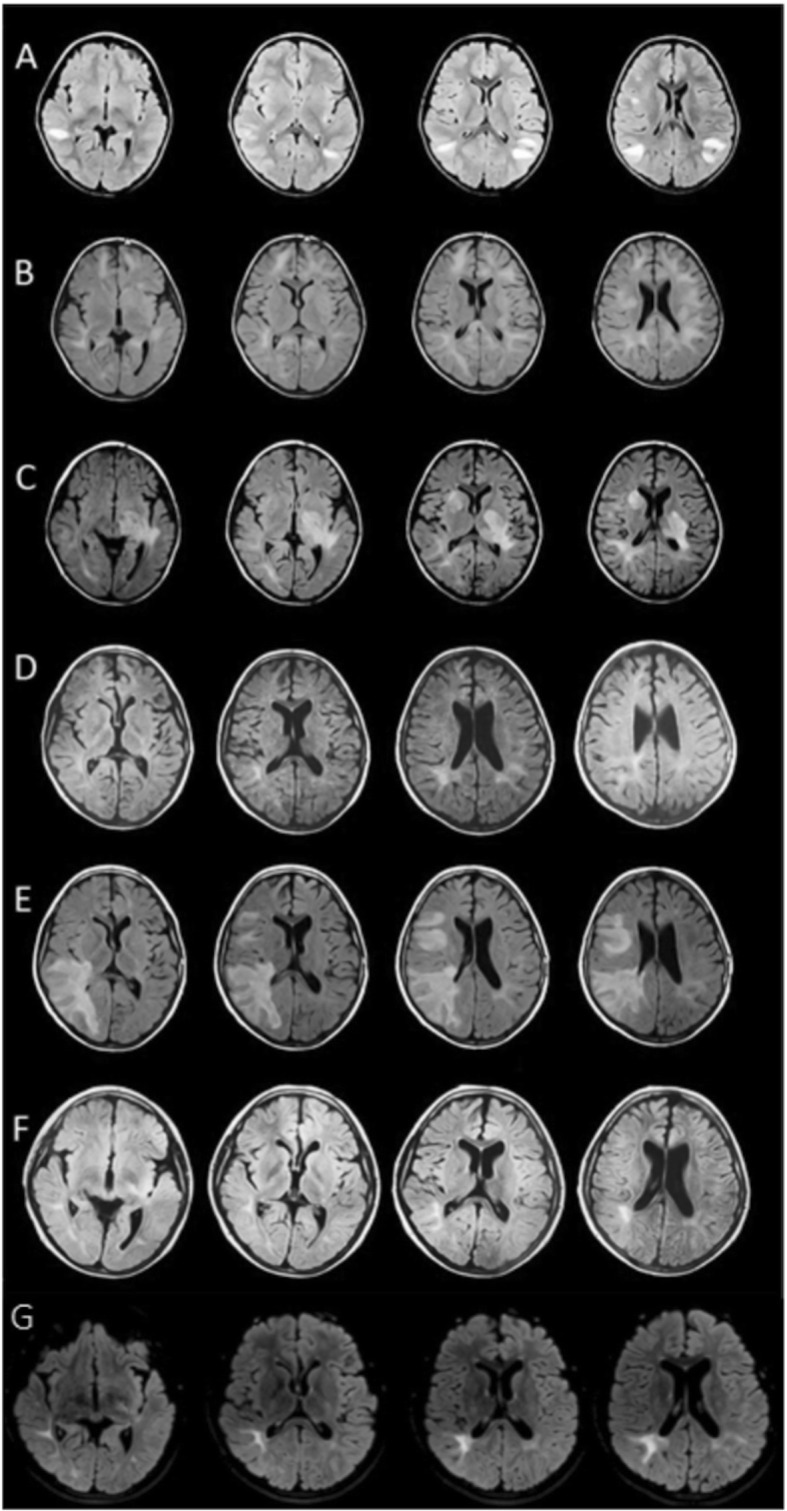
Fluid-attenuated inversion recovery T2 sequences showing multiple hyperintense lesions through the years. a 2008: Multiple injuries, mainly subcortical in temporal and occipitotemporal gyrus, and in the white matter of the corona radiata and semioval center. Lesions are enhanced after contrast. b 2010: Frontal subcortical injuries. Periatrial and parahippocampal lesions. There is a persistence of white matter lesions, and there are new infratentorial lesions. c 2011: Persistence of the periatrial lesions and left midbrain lesion. d 2013: Persistence of periatrial lesions and two small and new lesions in the basal ganglia infratentorial lesions. e 2014: New appearance of lesions in the cortex. Frontal and right superior temporal subcortical lesions. Right periatrial lesion has increased in size, reaching the cortex, parietal and occipital gyrus and corona radiata. f 2015: Persistence of periatrial lesions. There are smaller lesions in the white matter of the middle and lower right temporal gyrus and the semioval center. g 2017: After 7 months with intravenous immunoglobulin treatment – same lesions as the last magnetic resonance imaging in 2015. No new changes
